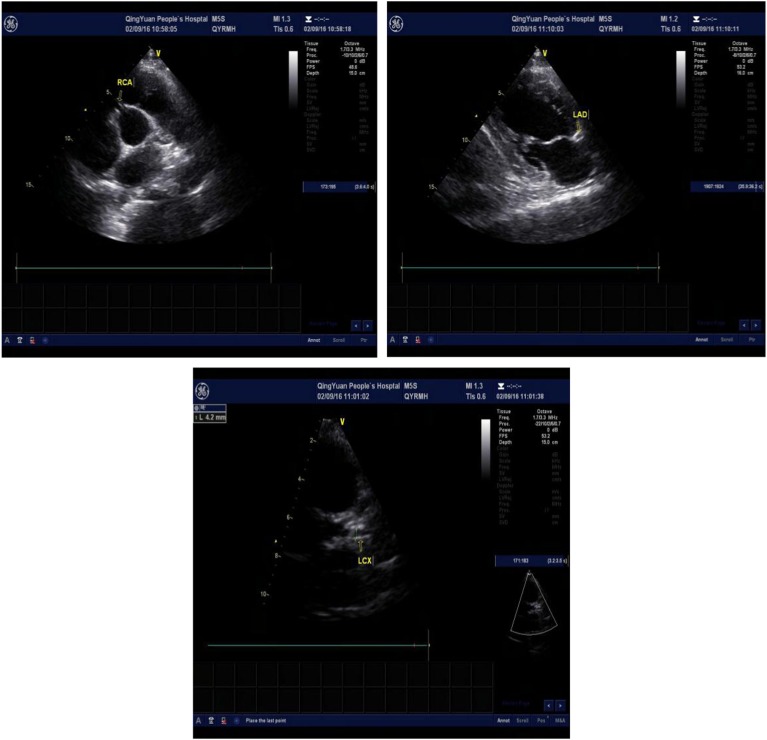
Echocardiography shows the dilated left coronary artery and right coronary artery (RCA) (RCA 4.6 mm, left anterior descending artery (LAD) 6.6 mm, left circumflex artery (LCX) 4.2 mm).
